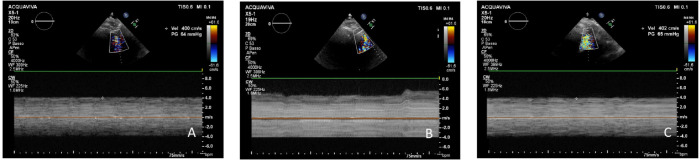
Transthoracic echocardiographic recordings of continuous-wave Doppler flow velocity signals in the same patient. Normal control (A), during left ventricular assist device thrombosis (B), and after thrombolysis (C). Note the intermittent oscillation of flow signal in B due to thrombotic obstruction. No oscillations of the flow signal are present in A and C.
